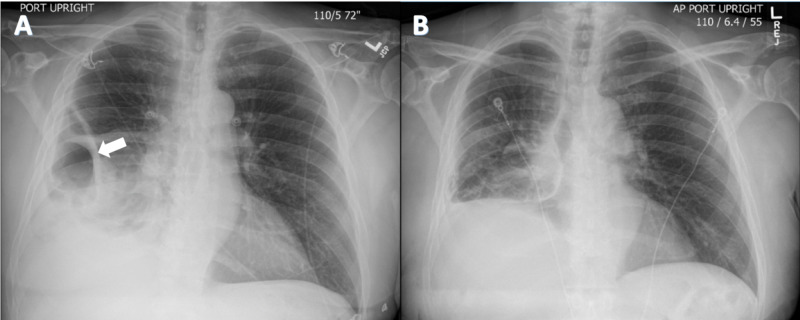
Chest X-ray. (A) Chest X-ray on presentation showed loculated fluid collection (arrow) with associated atelectasis at the right lung base. (B) Postoperative interval improvement in aeration of the right lung base. Right hemi-diaphragm remained persistently elevated with trace right pleural effusion and adjacent atelectasis.
